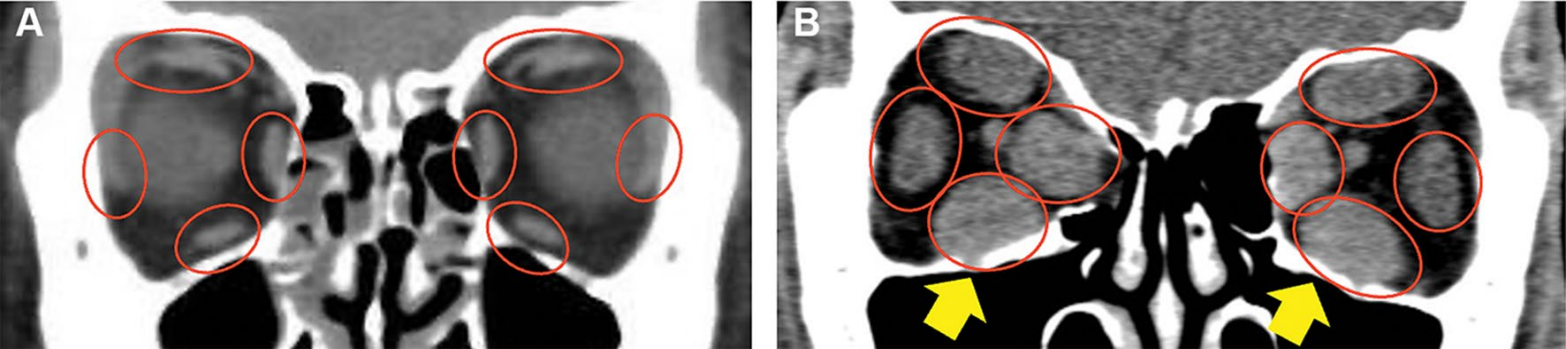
Representative images of extraocular muscles in normal (A) and TED (B). Red hollow circles indicate extraocular muscles in the human orbit. (A) Normal extraocular muscles shown in the coronal view in orbit CT are relatively flat. (B) Extraocular muscles in TED are thick and clubby compared with normal extraocular muscles, which are called extraocular muscle enlargements. In general, extraocular muscle enlargements in TED start at the inferior rectus muscles (yellow arrows).
